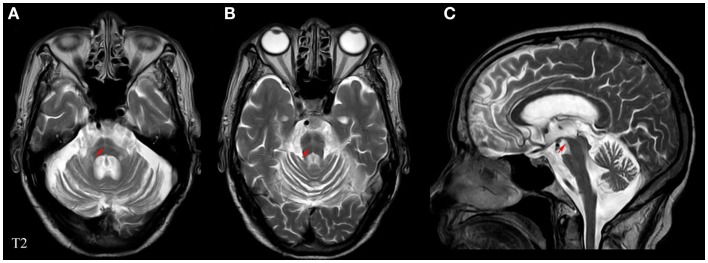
Representative MRI images of a patient with MSA. (A) Horizontal and (B) vertical hyperintensities in the pontine also known as “cross sign,” are shown in T2-weighted images. (C) Angle sign in the brainstem shown in a T2-weighted image. Red arrows indicate abnormalities pertaining to MSA.
